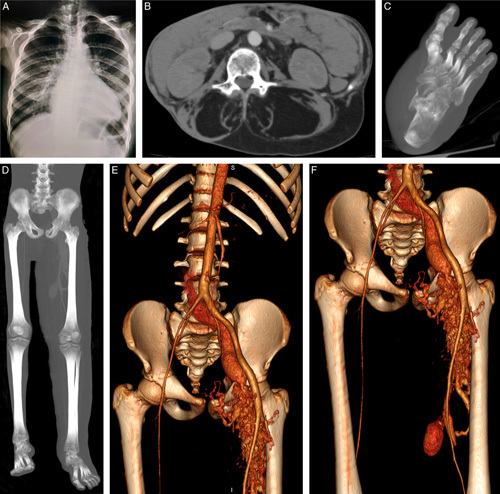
X-ray and contrast-enhanced computed tomography findings. (A) Posteroanterior chest x-ray showing mild lateral scoliosis. (B) Axial view in the arterial phase showcasing two nonenhancing lipomatous masses in the trunk replacing the paraspinal muscles. (C) Maximum intensity projection view of the left foot showing sandal gap deformity with increased soft tissue density between the first and second toes. (D) Maximum intensity projection view of the lower limbs demonstrating the severe overgrowth with leg-length discrepancy of 5 cm. (E and F) Three-dimensional reconstruction of computed tomography angiography in the arterial phase showing arteriovenous fistulas with venous malformations and a superficial femoral vein aneurysm.
